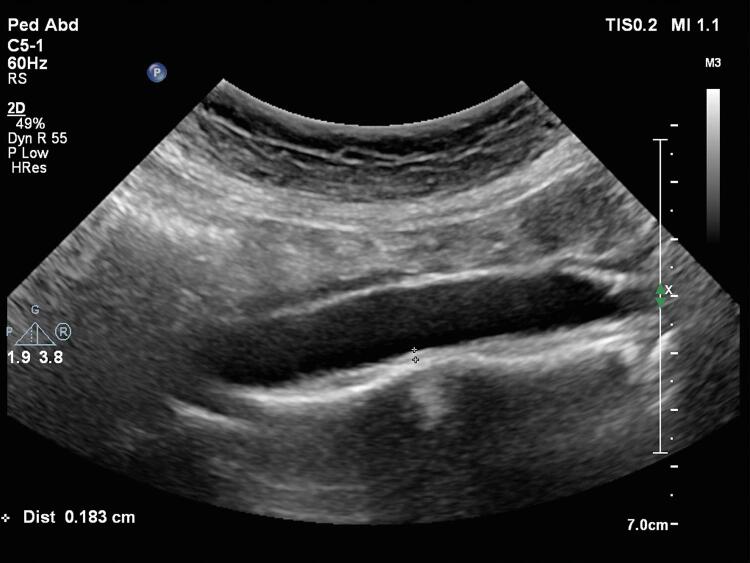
Medida da espessura médio-intimal da artéria aorta abdominal por ultrassonografia modo B mostrando valor aumentado (1,83 mm) em um paciente com talassemia menor.
